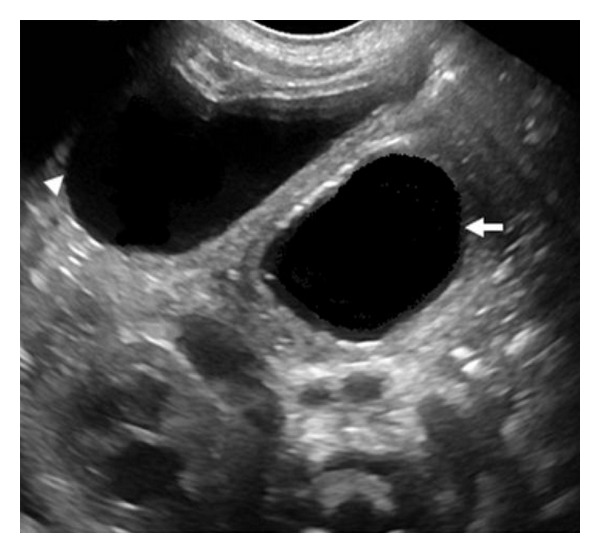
Transverse ultrasound of the pelvis shows a large utricular cyst in the midline (white arrow), posterior to the bladder (white arrowhead).
